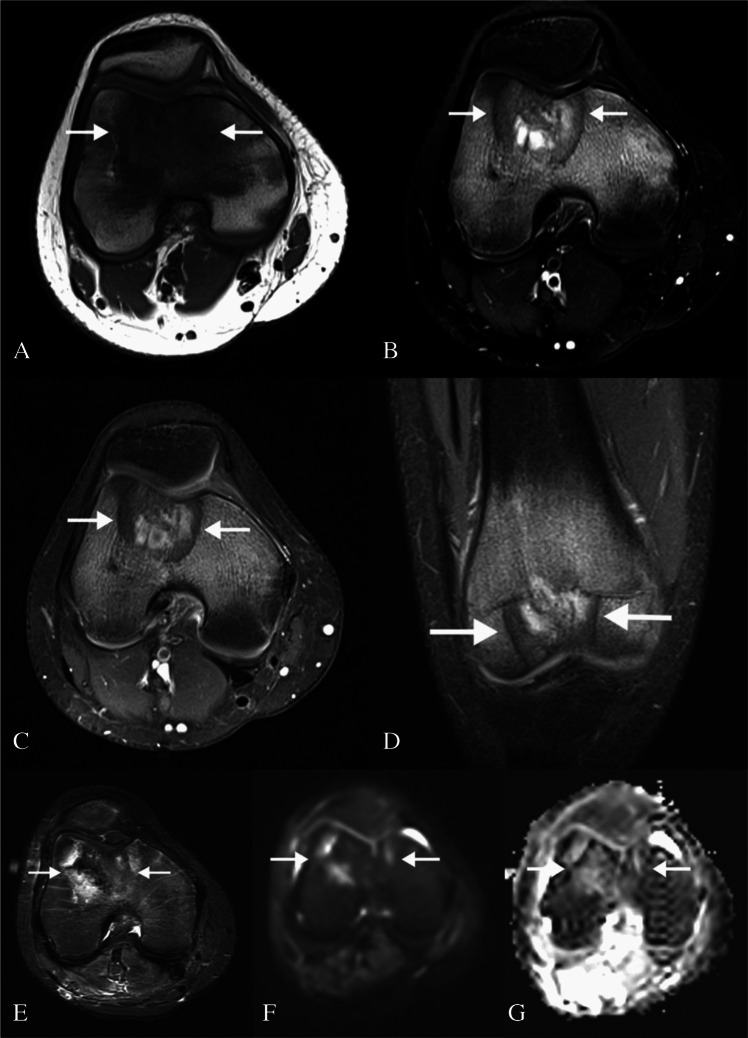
14-year-old female with Ewing sarcoma of the distal femoral epiphysis. Axial T1 (A) and STIR (B) and axial (C) and coronal (D) T1 FS post contrast MRI images demonstrate low to intermediate T1 signal, variable intermediate to high T2 signal, and heterogeneous enhancement with marked surrounding edema without an associated soft tissue mass. Axial STIR (E), DWI (F), and ADC map (G) images after radiation treatment demonstrate decreased T2 hyperintensity with few regions of T2 shine through but no restricted diffusion
